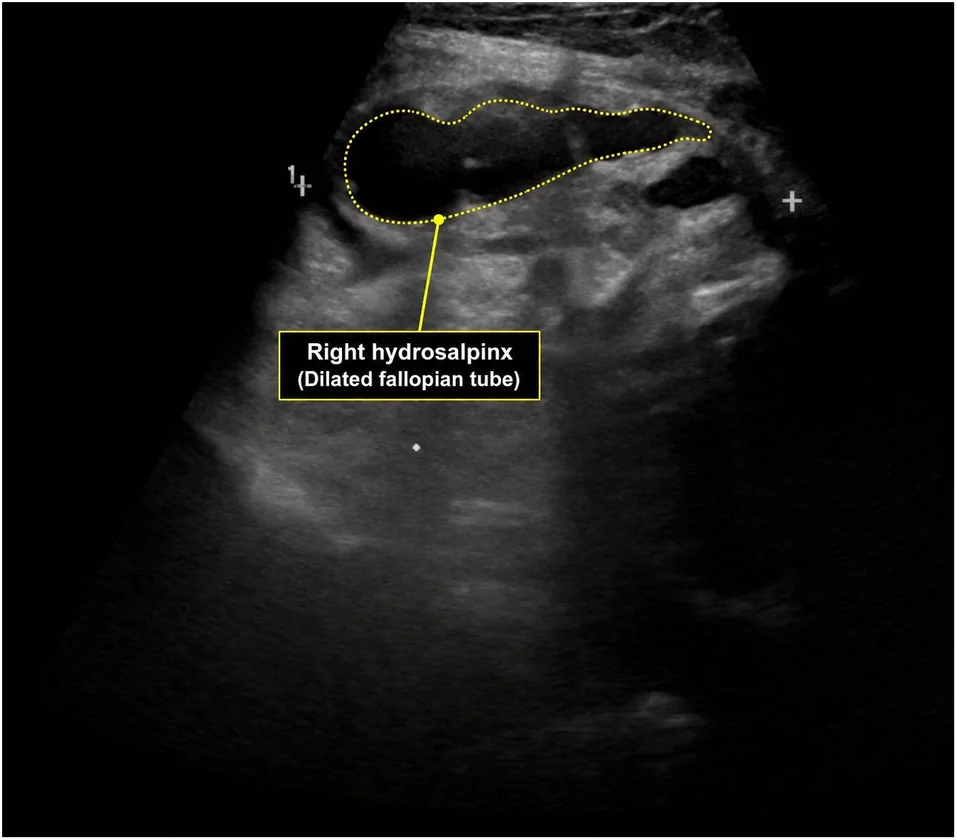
Transabdominal pelvic ultrasonography demonstrating a right hydrosalpinx with dilatation of the fallopian tube and an anechoic cystic tubular structure within the right adnexal region.
